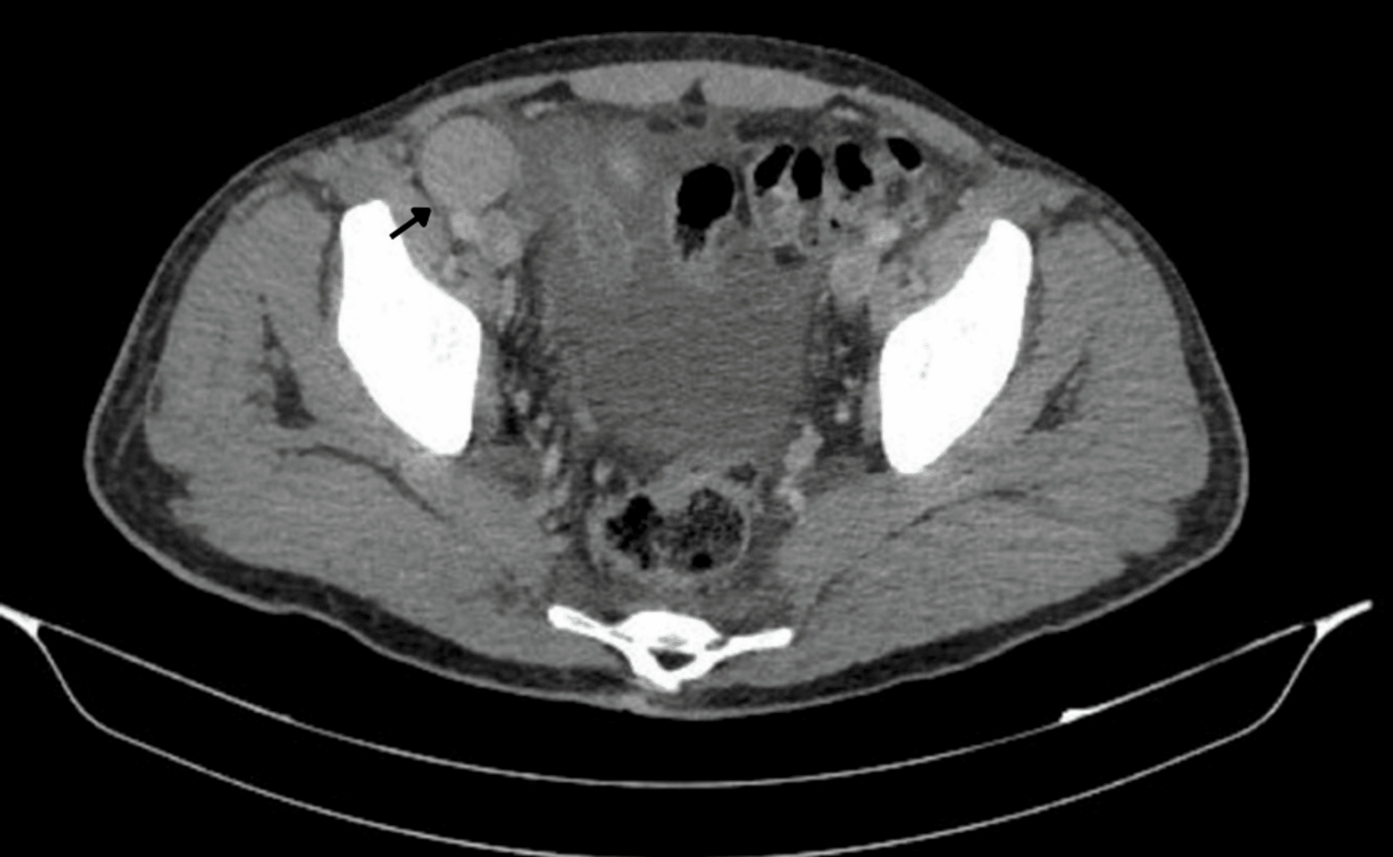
Contrast-enhanced CT of the pelvis, axial plane. Axial CT image of the pelvis demonstrating enlarged iliac and inguinal lymph nodes. The arrow indicates pathological lymphadenopathy consistent with metastatic involvement. CT: computed tomography
